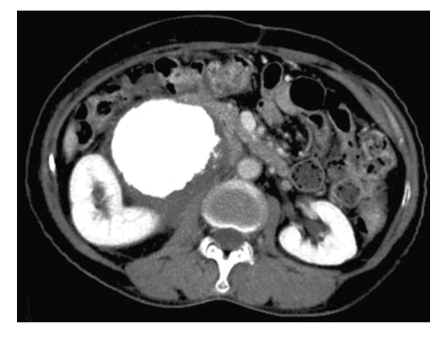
Abdominal CT revealed a large mass with diffuse calcification in the right retroperitoneal space.
